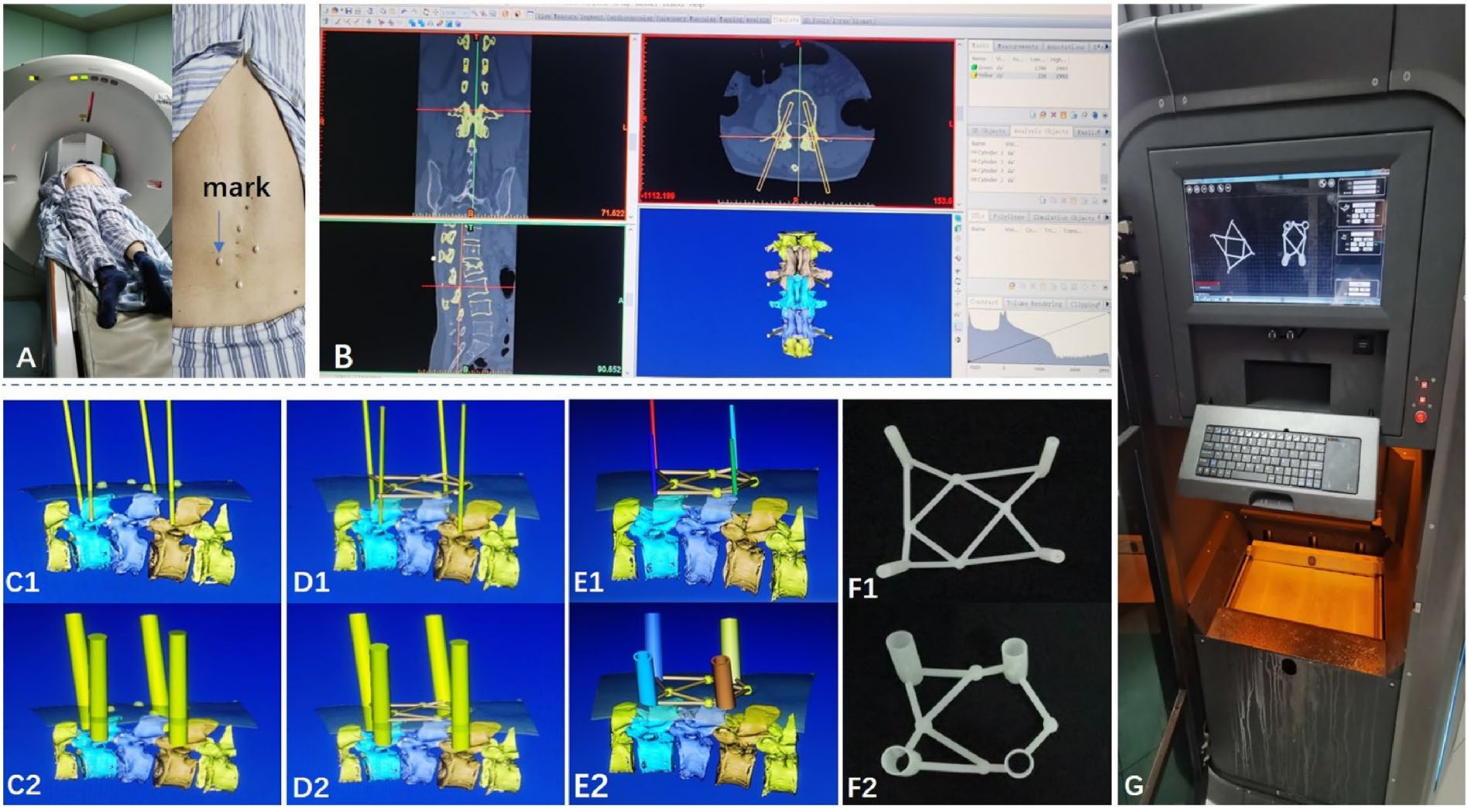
The main process of percutaneous guide plate design and 3D printing. (A) Preoperative localization of markers was performed by CT scan. (B–E) CAD of the main process of personalized percutaneous guide plate. (F, G) 3D printer is printing the real percutaneous guide plate.
